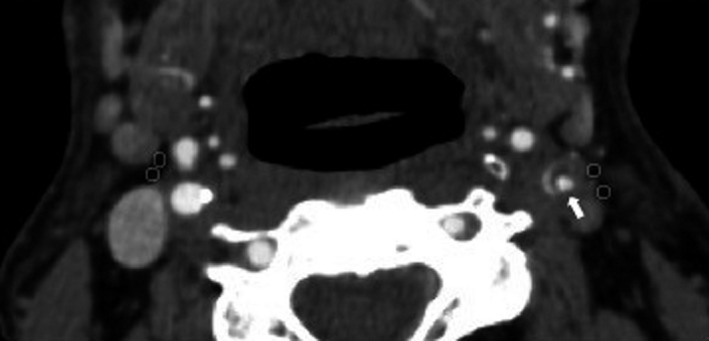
Computed tomography angiography image of a 72‐year‐old man presenting with a left middle cerebral artery territory infarction. Two regions‐of‐interest (ROI) were placed in the perivascular fat surrounding the stenotic (arrow) left internal cerebral artery (ICA) and 2 ROIs were placed in the perivascular fat surrounding the nonstenotic right ICA on the same axial slice. In this case, the 2 left ROIs were −65.3 and −62.8 and the 2 right ROIs were −80.3 and −78.9.
